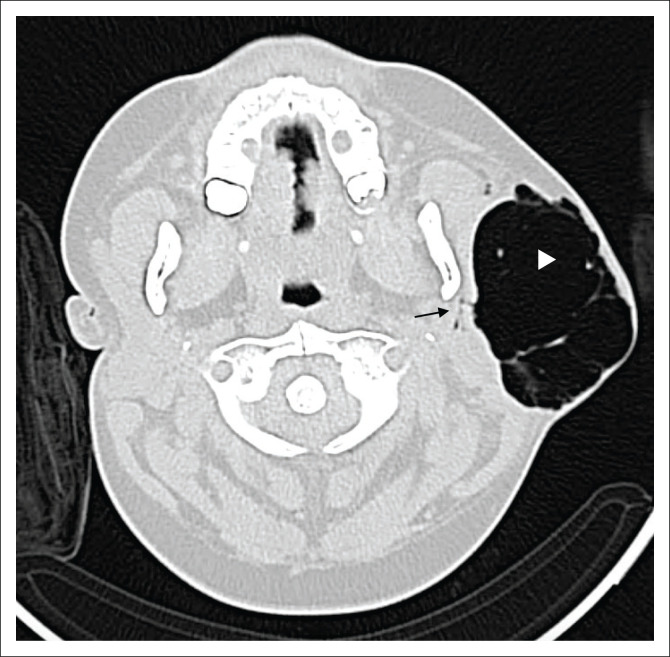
Axial non-contrasted computed tomography demonstrates a large, multiseptated focal collection of gas in the subcutaneous tissue overlying the left parotid gland (white arrowhead). Underlying communication with branching gas pattern in the ductal system of the left parotid gland is shown (black arrow). No gas is present in the parapharyngeal fat space or deep neck spaces.
